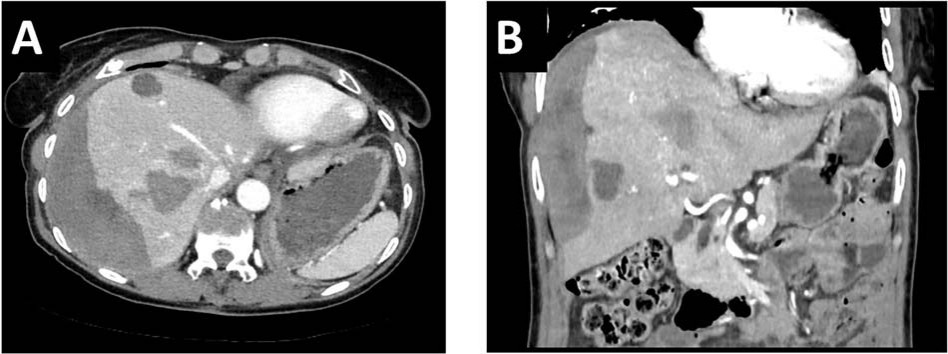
(A/B) CT-scan showing a large intrahepatic hematoma after liver biopsy on the right side.
